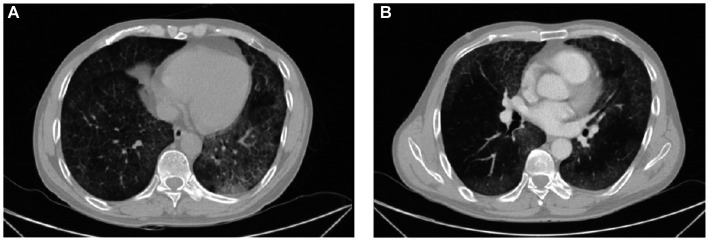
HRCT images. (A,B) Ground glass opacity and patchy shadow on bilateral lung, showing a crazy-paving pattern, mainly in the lower lobes.
